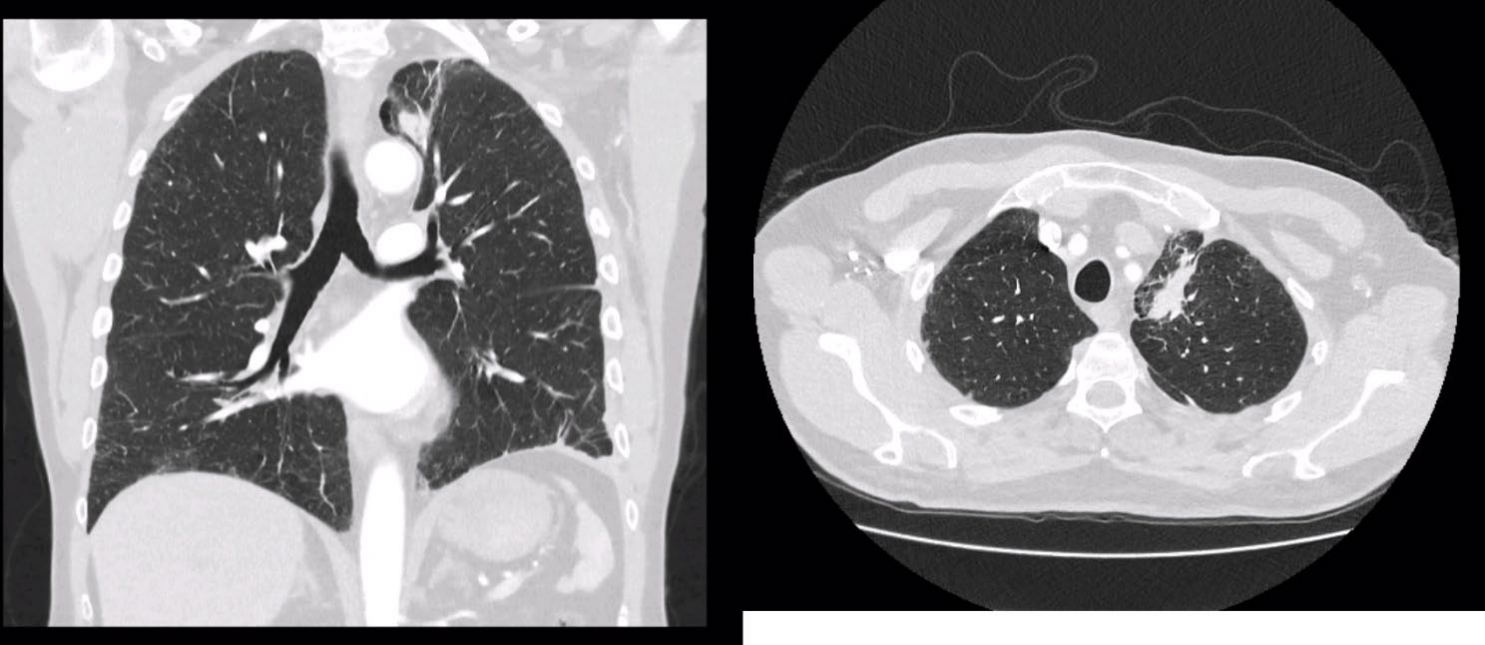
Shows coronal (left) and sagittal (right) views of the CT thorax taken post-operatively. Post-surgical changes can be appreciated in the left lung with no evidence of tumour
